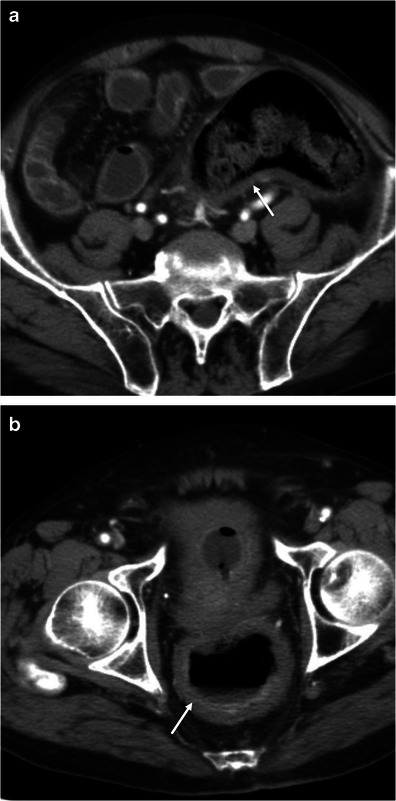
Ischaemic colitis. Axial contrast-enhanced CT scans through the descending colon (a) and rectum (b) show the thickened hypoattenuating bowel wall due to ischaemia. The diagnosis was confirmed with colonoscopy and biopsy
